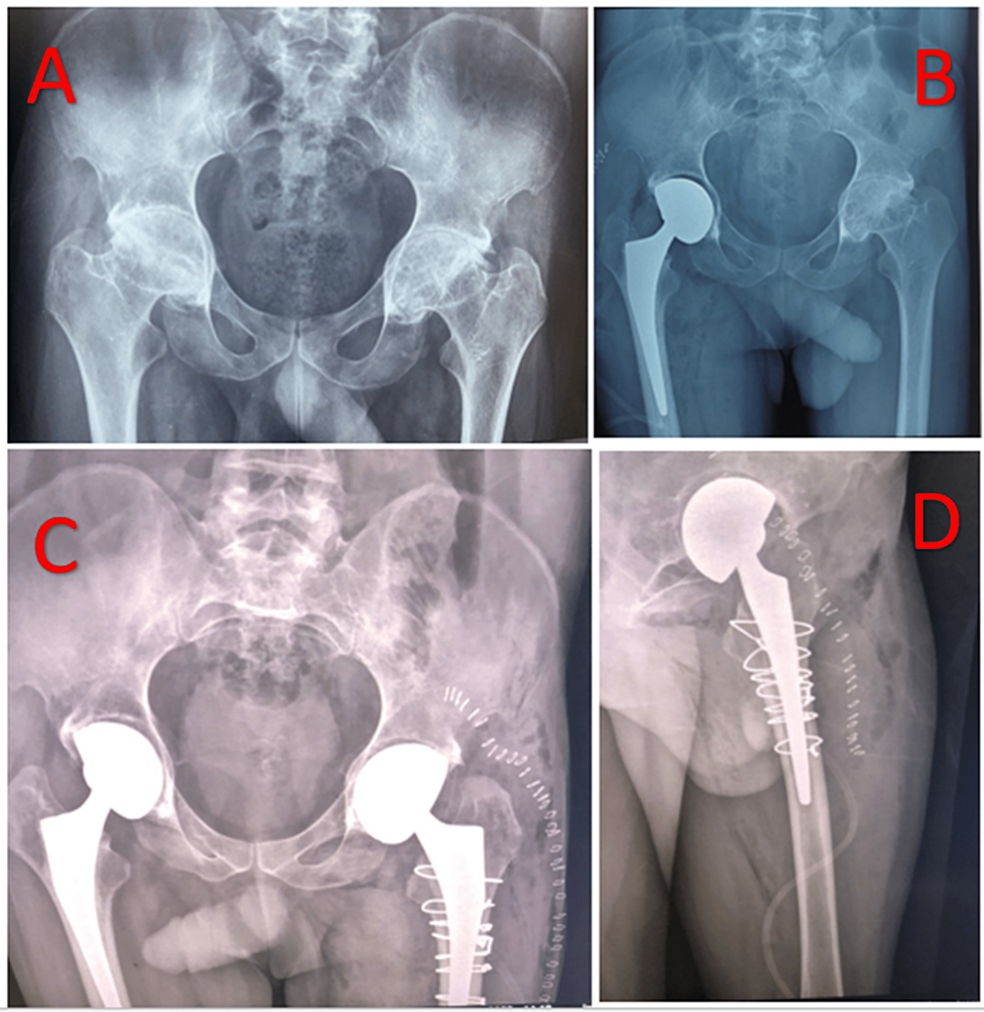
Radiographic assessment of case 1 A: X-ray of the pelvis showing bilateral coxarthritis at the ankylosis stage. B: Control radiograph after the right prosthesis had been fitted. C: pelvis X-ray after completion of the left prosthesis, complicated intraoperatively by a peri-prosthetic fracture treated by steel wire cerclage. D: Immediate postoperative hip X-ray, profile view.
